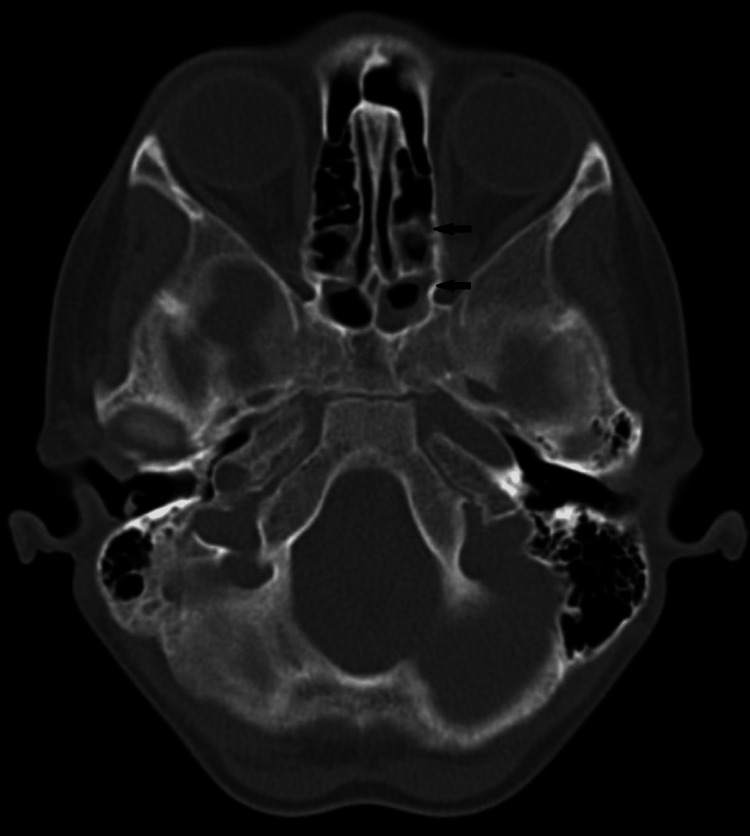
Axial CT scan showing partial mucosal thickening of ethmoid and sphenoid sinuses (black arrow) CT: computed tomography
